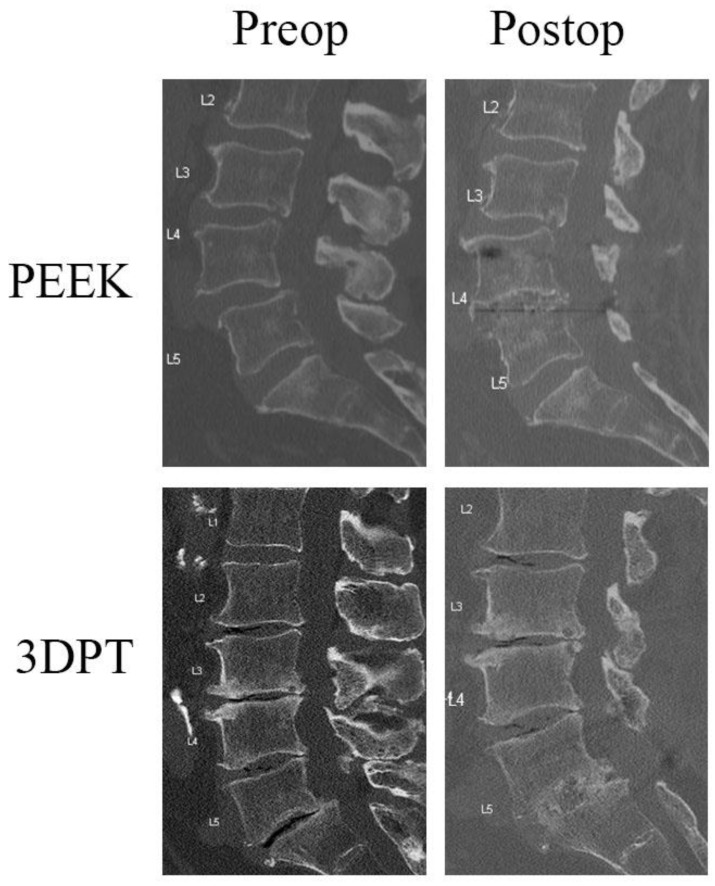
Representative preoperative and postoperative images of patients who underwent lumbar interbody fusion with either PEEK or 3DPT cage. Patient 1’s preoperative sagittal CT demonstrating L4–5 grade 1 spondylolisthesis and postoperative CT of L4–5 PLIF with PEEK implant at 3 years postoperatively. Patient 2’s preoperative sagittal CT with L5-S1 grade 1 spondylolisthesis, and 2-year postoperative CT of L5-S1 PLIF with 3DPT implant.
